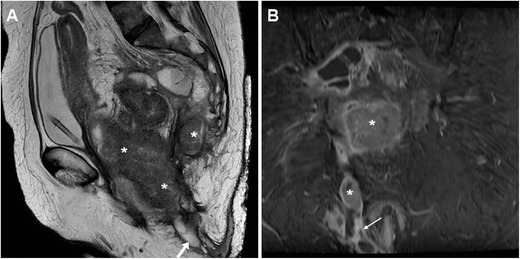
Locally advanced rectal cancer with superinfection and ECF formation in a 54-year-old woman with septic fever. T2-weighted MRI images (A) showed a large solid tumour (*) invading the vagina and perineum. Additionally, a 1-cm wide fluid-containing ECF (arrows) coursed postero-inferiorly towards the perianal skin and showed peripheral enhancement (thin arrow) on post-gadolinium fat-suppressed T1-weighted acquisition (B)
